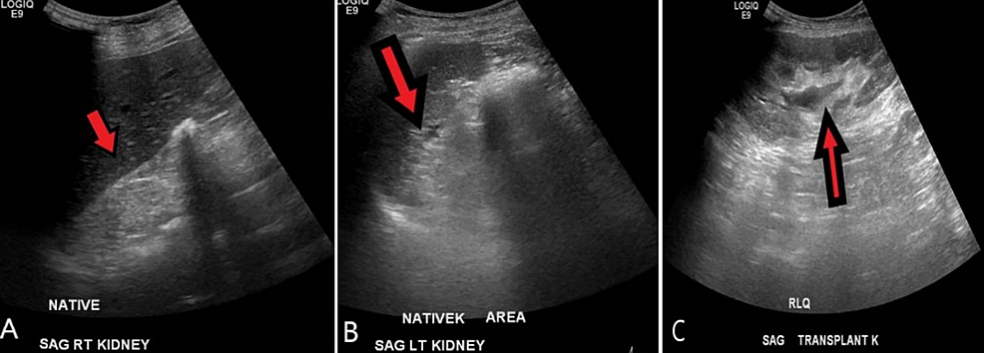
Ultrasound of native and transplanted kidneys with Doppler imaging. A real-time sonography of the kidneys and bladder was performed. The native kidneys are markedly atrophic and difficult to visualize (A: sagittal view of the right native kidney; B: sagittal view of the left native kidney). The transplant kidney measures 12.5 cm in size (C: sagittal view of the transplant kidney in the right lower quadrant).  No hydronephrosis, shadowing stone, or mass lesion is identified. The resistive indices are within normal limits.  Red arrows identify the kidney.
